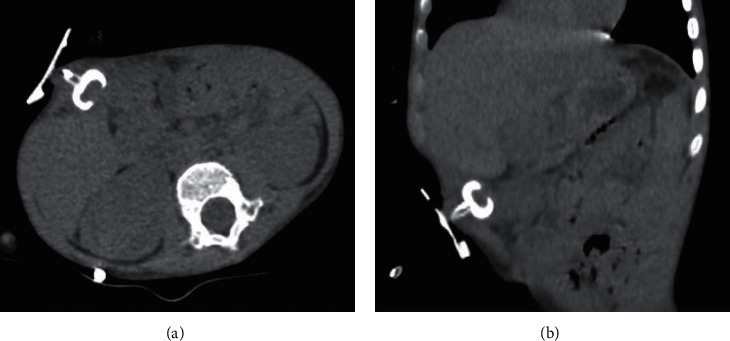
In transverse cut (a) and coronal cut (b) of CT exam, the gastrostomy tube was correctly positioned in the gastric antrum pulled to the right.
